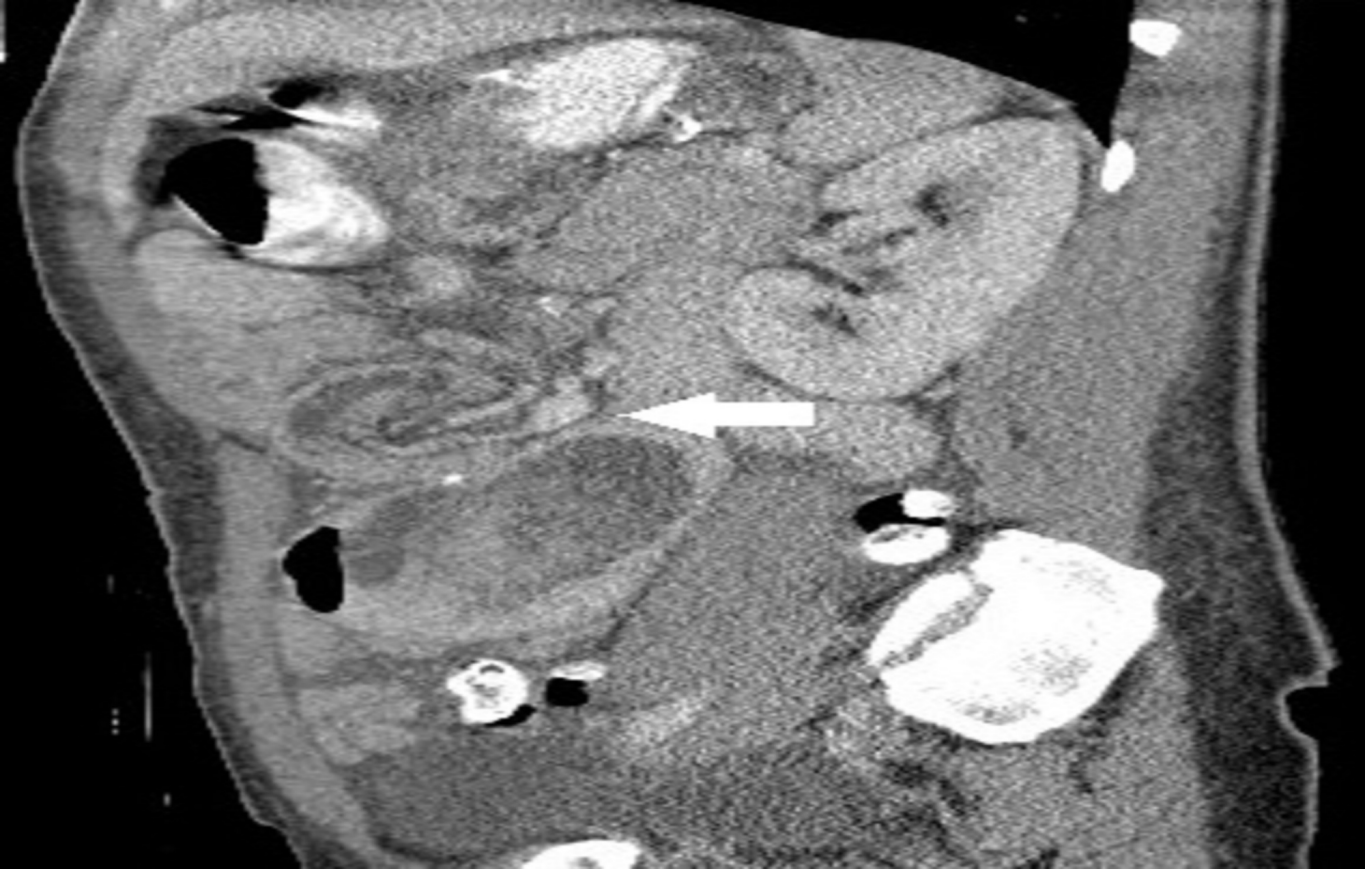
Abdominal computed tomography (CT) scan, sagittal view, showing dilatation of the biliopancreatic limb of the REYGB with SB intussusception (arrow) through the jejunojejunostomy REYGB: Roux-en-Y gastric bypass surgery; SB: small bowel.
